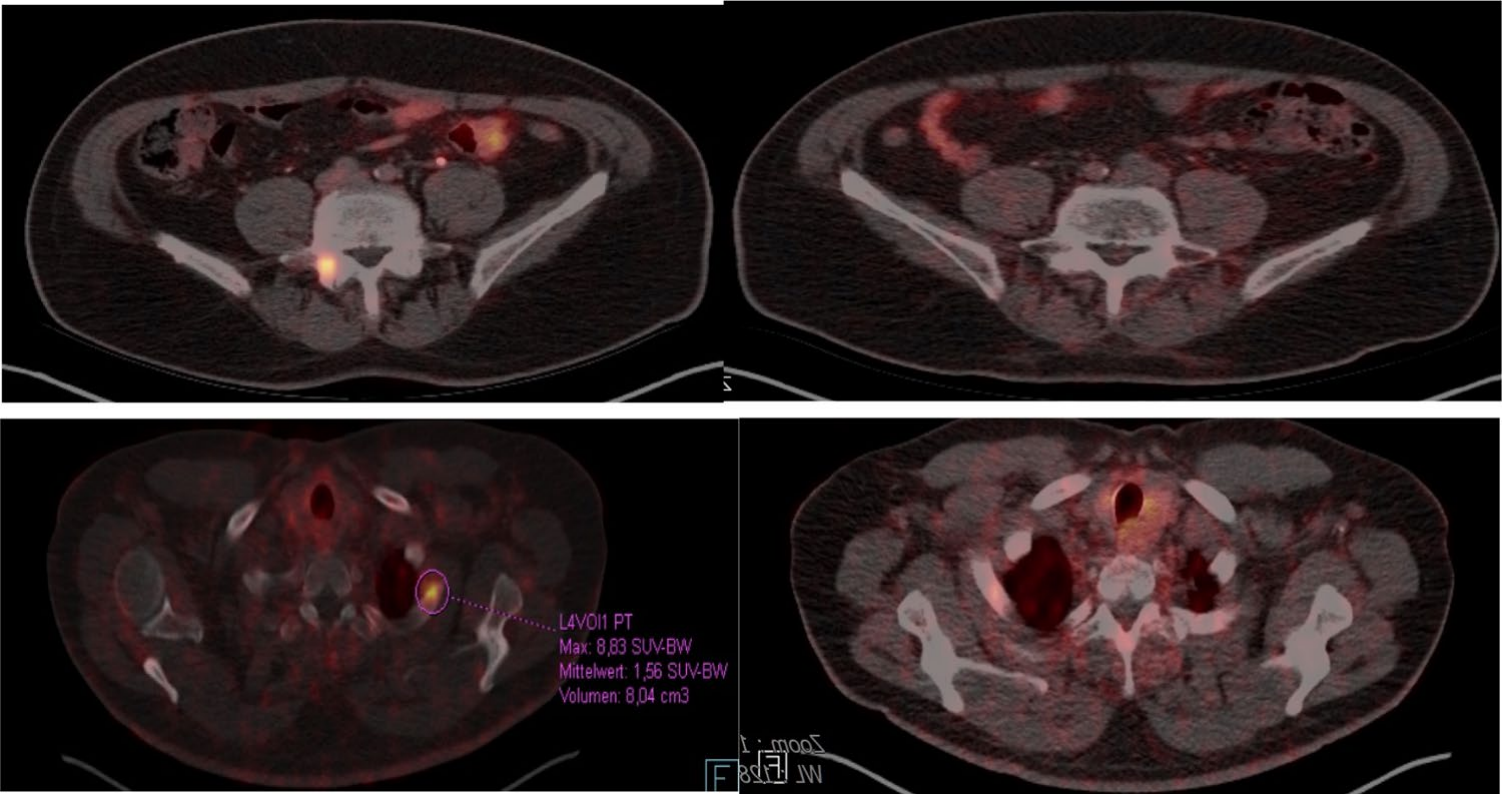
Example PSMA PET before and after SBRT
